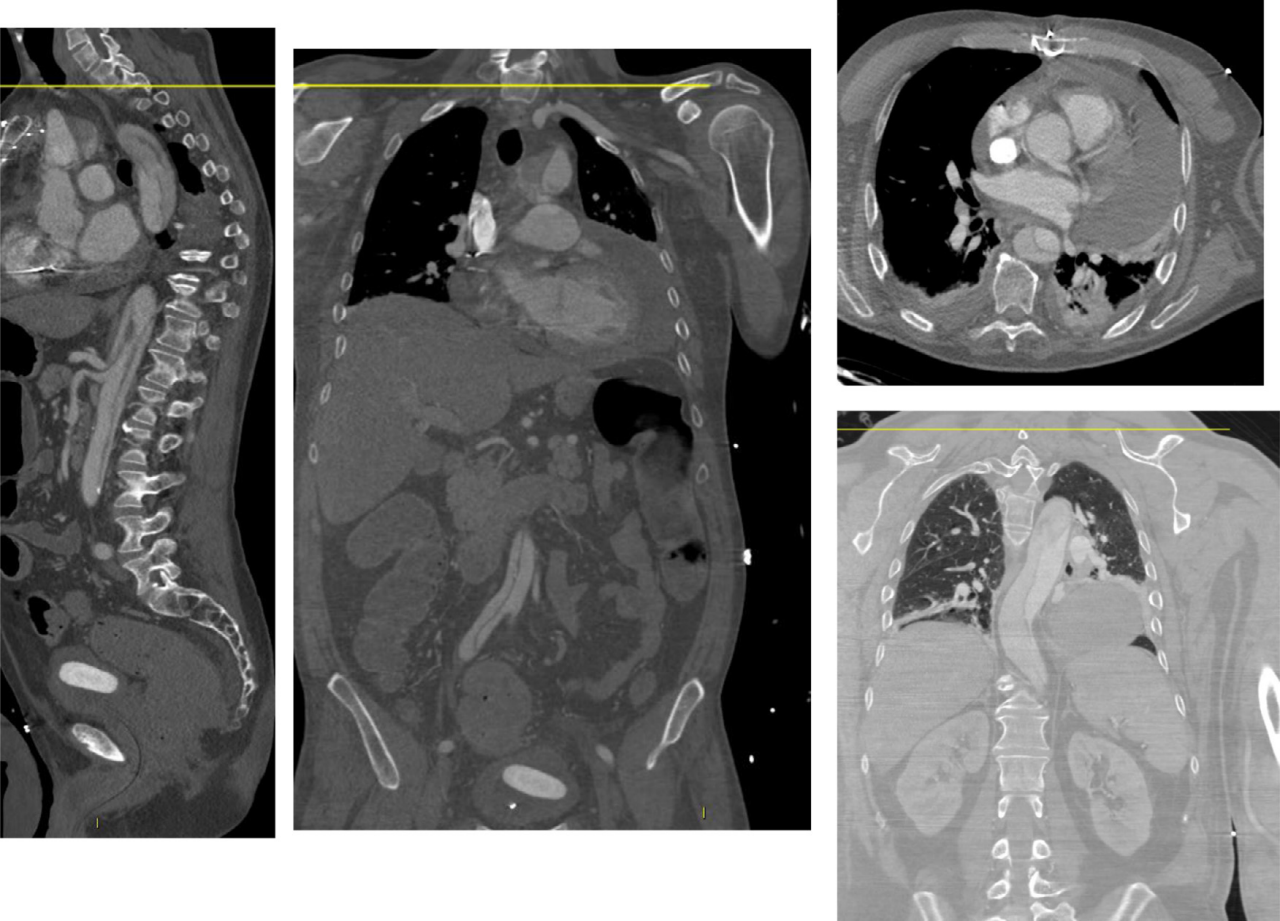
(A-D) Computed Tomography Angiography of the thoracic and abdominal cavities; sagittal, coronal and axial MPR reconstructions. Computed Tomography Angiography had confirmed the presence of a thin intraluminal hypodense line (intimal flap) in the thoracic aorta, and so had identified the presence of a suggestive dissection of the thoracic aorta at the ascending-arch passage which extends caudally to the iliac bifurcation and right iliac artery to its bifurcation, involving entirely the abdominal aorta for its entire course. It was also identified a pericardial effusion layer with a maximum thickness of about 5 cm (at the anterior pericardial wall).
